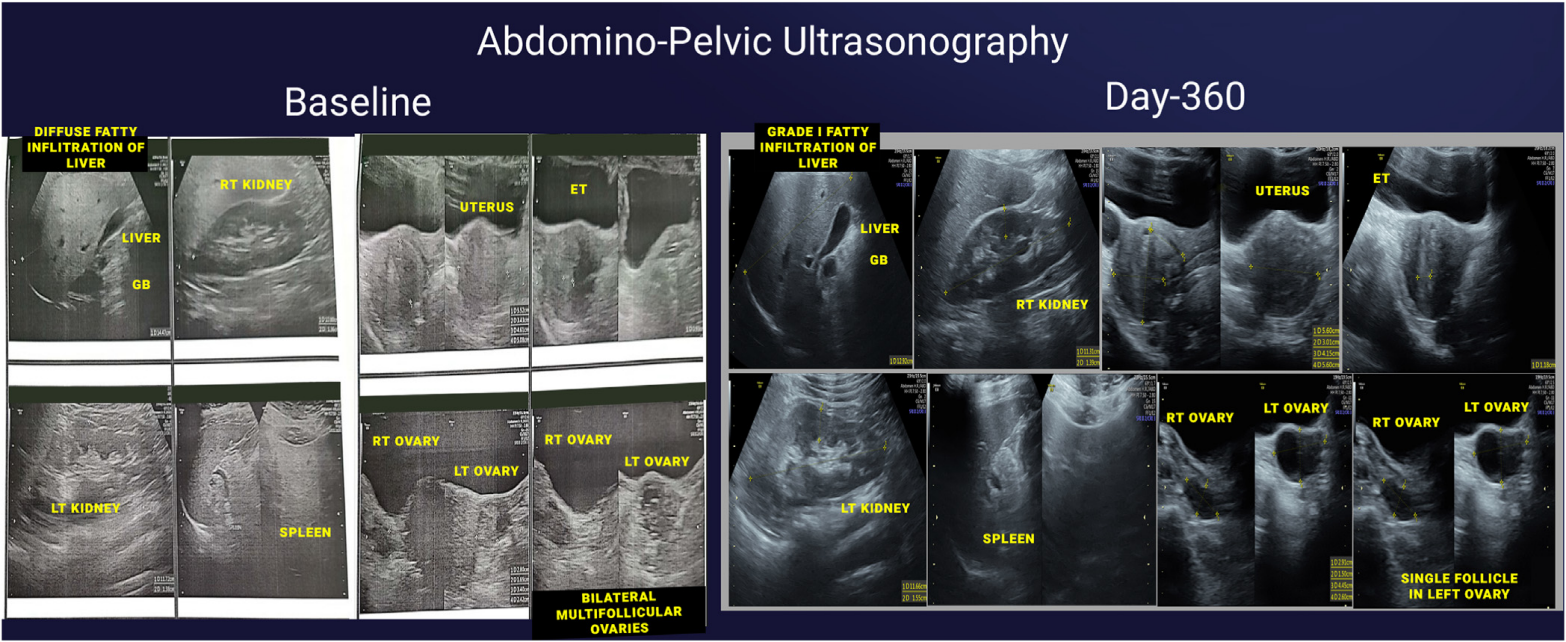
Abdominopelvic ultrasonography between baseline and day-360 baseline ultrasound revealed normal-sized ovaries with multiple follicles, consistent with PCOS: *right* ovary at 2.8 × 1.6 cm, *left* at 3.4 × 2.4 cm; a mildly bulky uterus; and diffuse fatty liver infiltration. After 360 days, *right* ovary measured 2.9 × 1.5 cm, *left* ovary 4.4 × 2.6 cm with a 23 × 19 mm follicle; and improvement to grade I fatty liver infiltration. Abbreviations: *ET* = endometrial thickness; *GB* = gall bladder; *LT* = *left*; *RT* = *right*.•Computed tomography ccan: Epicardial volume decreased by 21.8% (Fig. 3), pericardial volume by 69.9% (Fig. 4), and visceral fat by 44.4%.
